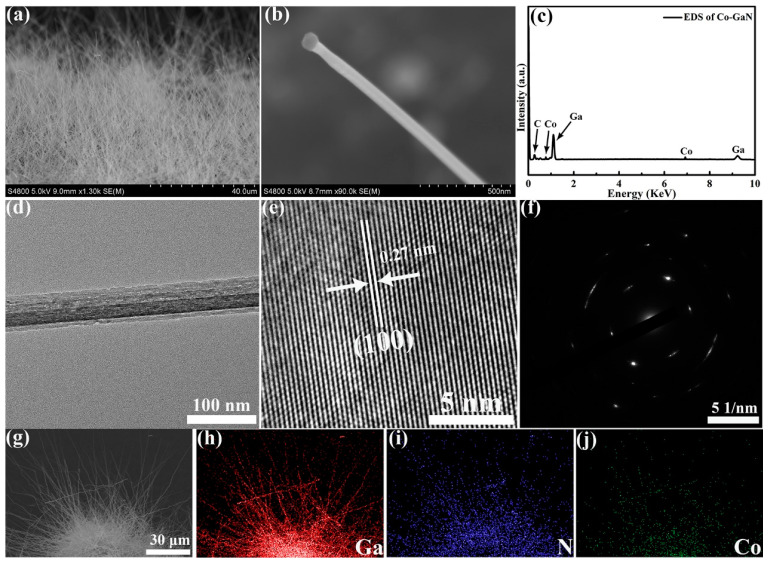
(a,b) SEM images at different magnifications. (c) Energy-dispersive X-ray (EDX) elemental test. (d) TEM image. (e) High-resolution TEM images. (f) SAED images. (g–j) EDS mapping analysis of Co-GaN.
